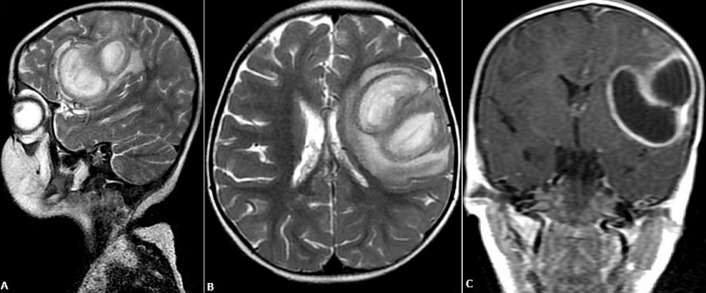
A) MRI brain (sagittal section) of head suggestive of mass in the front-tempero-parietal region; B) MRI brain (axial section) of brain suggestive of mass in the left lobe of the brain causing mass effect by doing a midline shift of 4mm; C) MRI brain (coronal section) showing a mass on the left side
